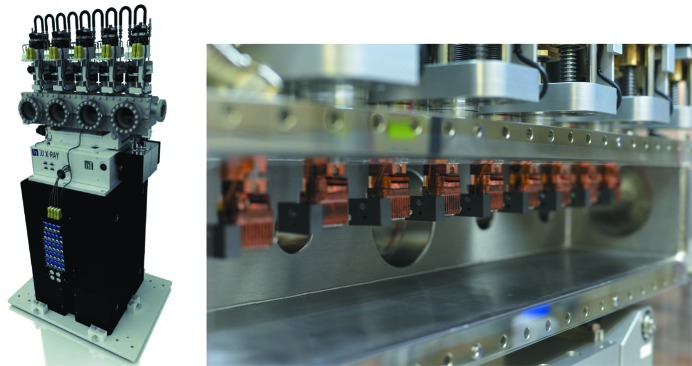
CAD-model of the JJ X-ray transfocator unit (left) and photograph of the lens cassettes inside the chamber installed in the XTD9 tunnel (right).
